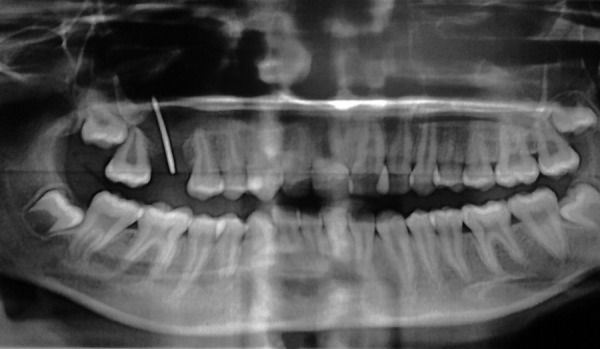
Orthopantomogram showing oroantral defect with gutta-percha placed in it
